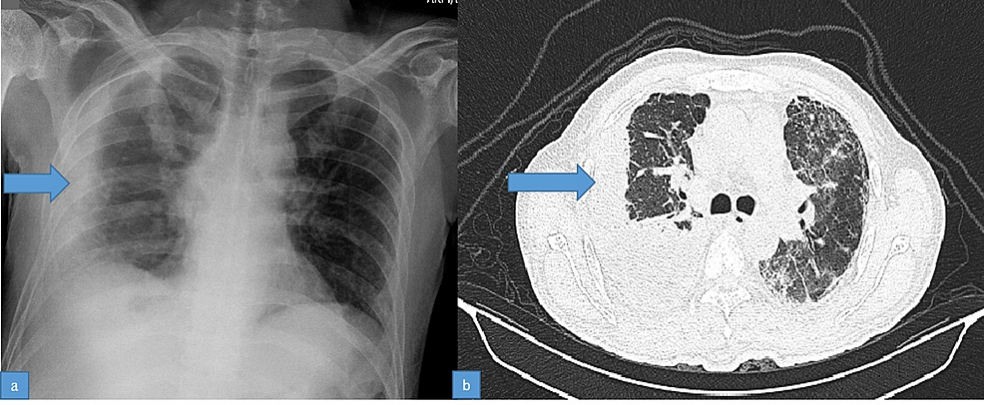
(a) Chest radiograph showing empyema on the right. (b) HRCT of the thorax showing right empyema. HRCT: high-resolution computed tomography
